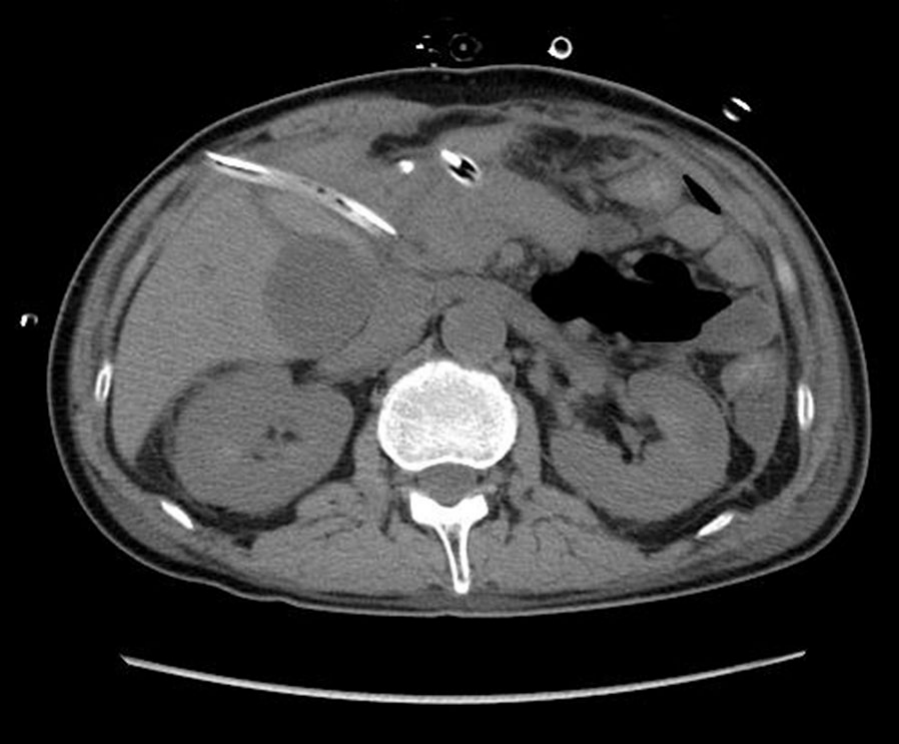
Computed tomography 6 days after the first emergency surgery. Fluid accumulation could be observed in the anterior area of the inferior duodenal flexure together with peritoneal hypertrophy of the whole abdominal area
